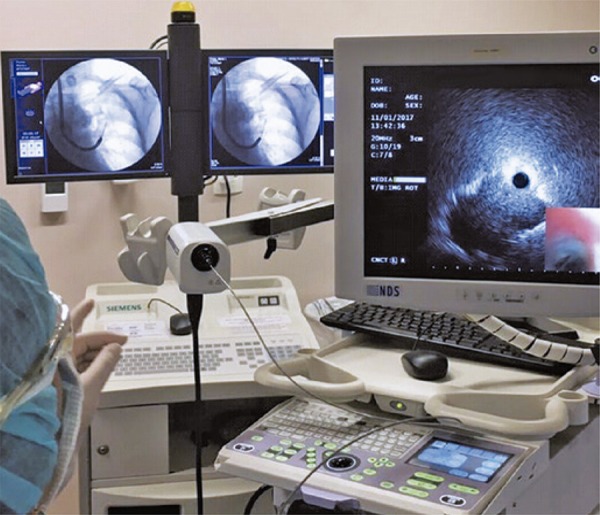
Bronchoscopy with radial endobronchial ultrasound and fluoroscopy for better visualization and performance of transbronchial biopsy of the pulmonary nodule in the upper left lung lobe
